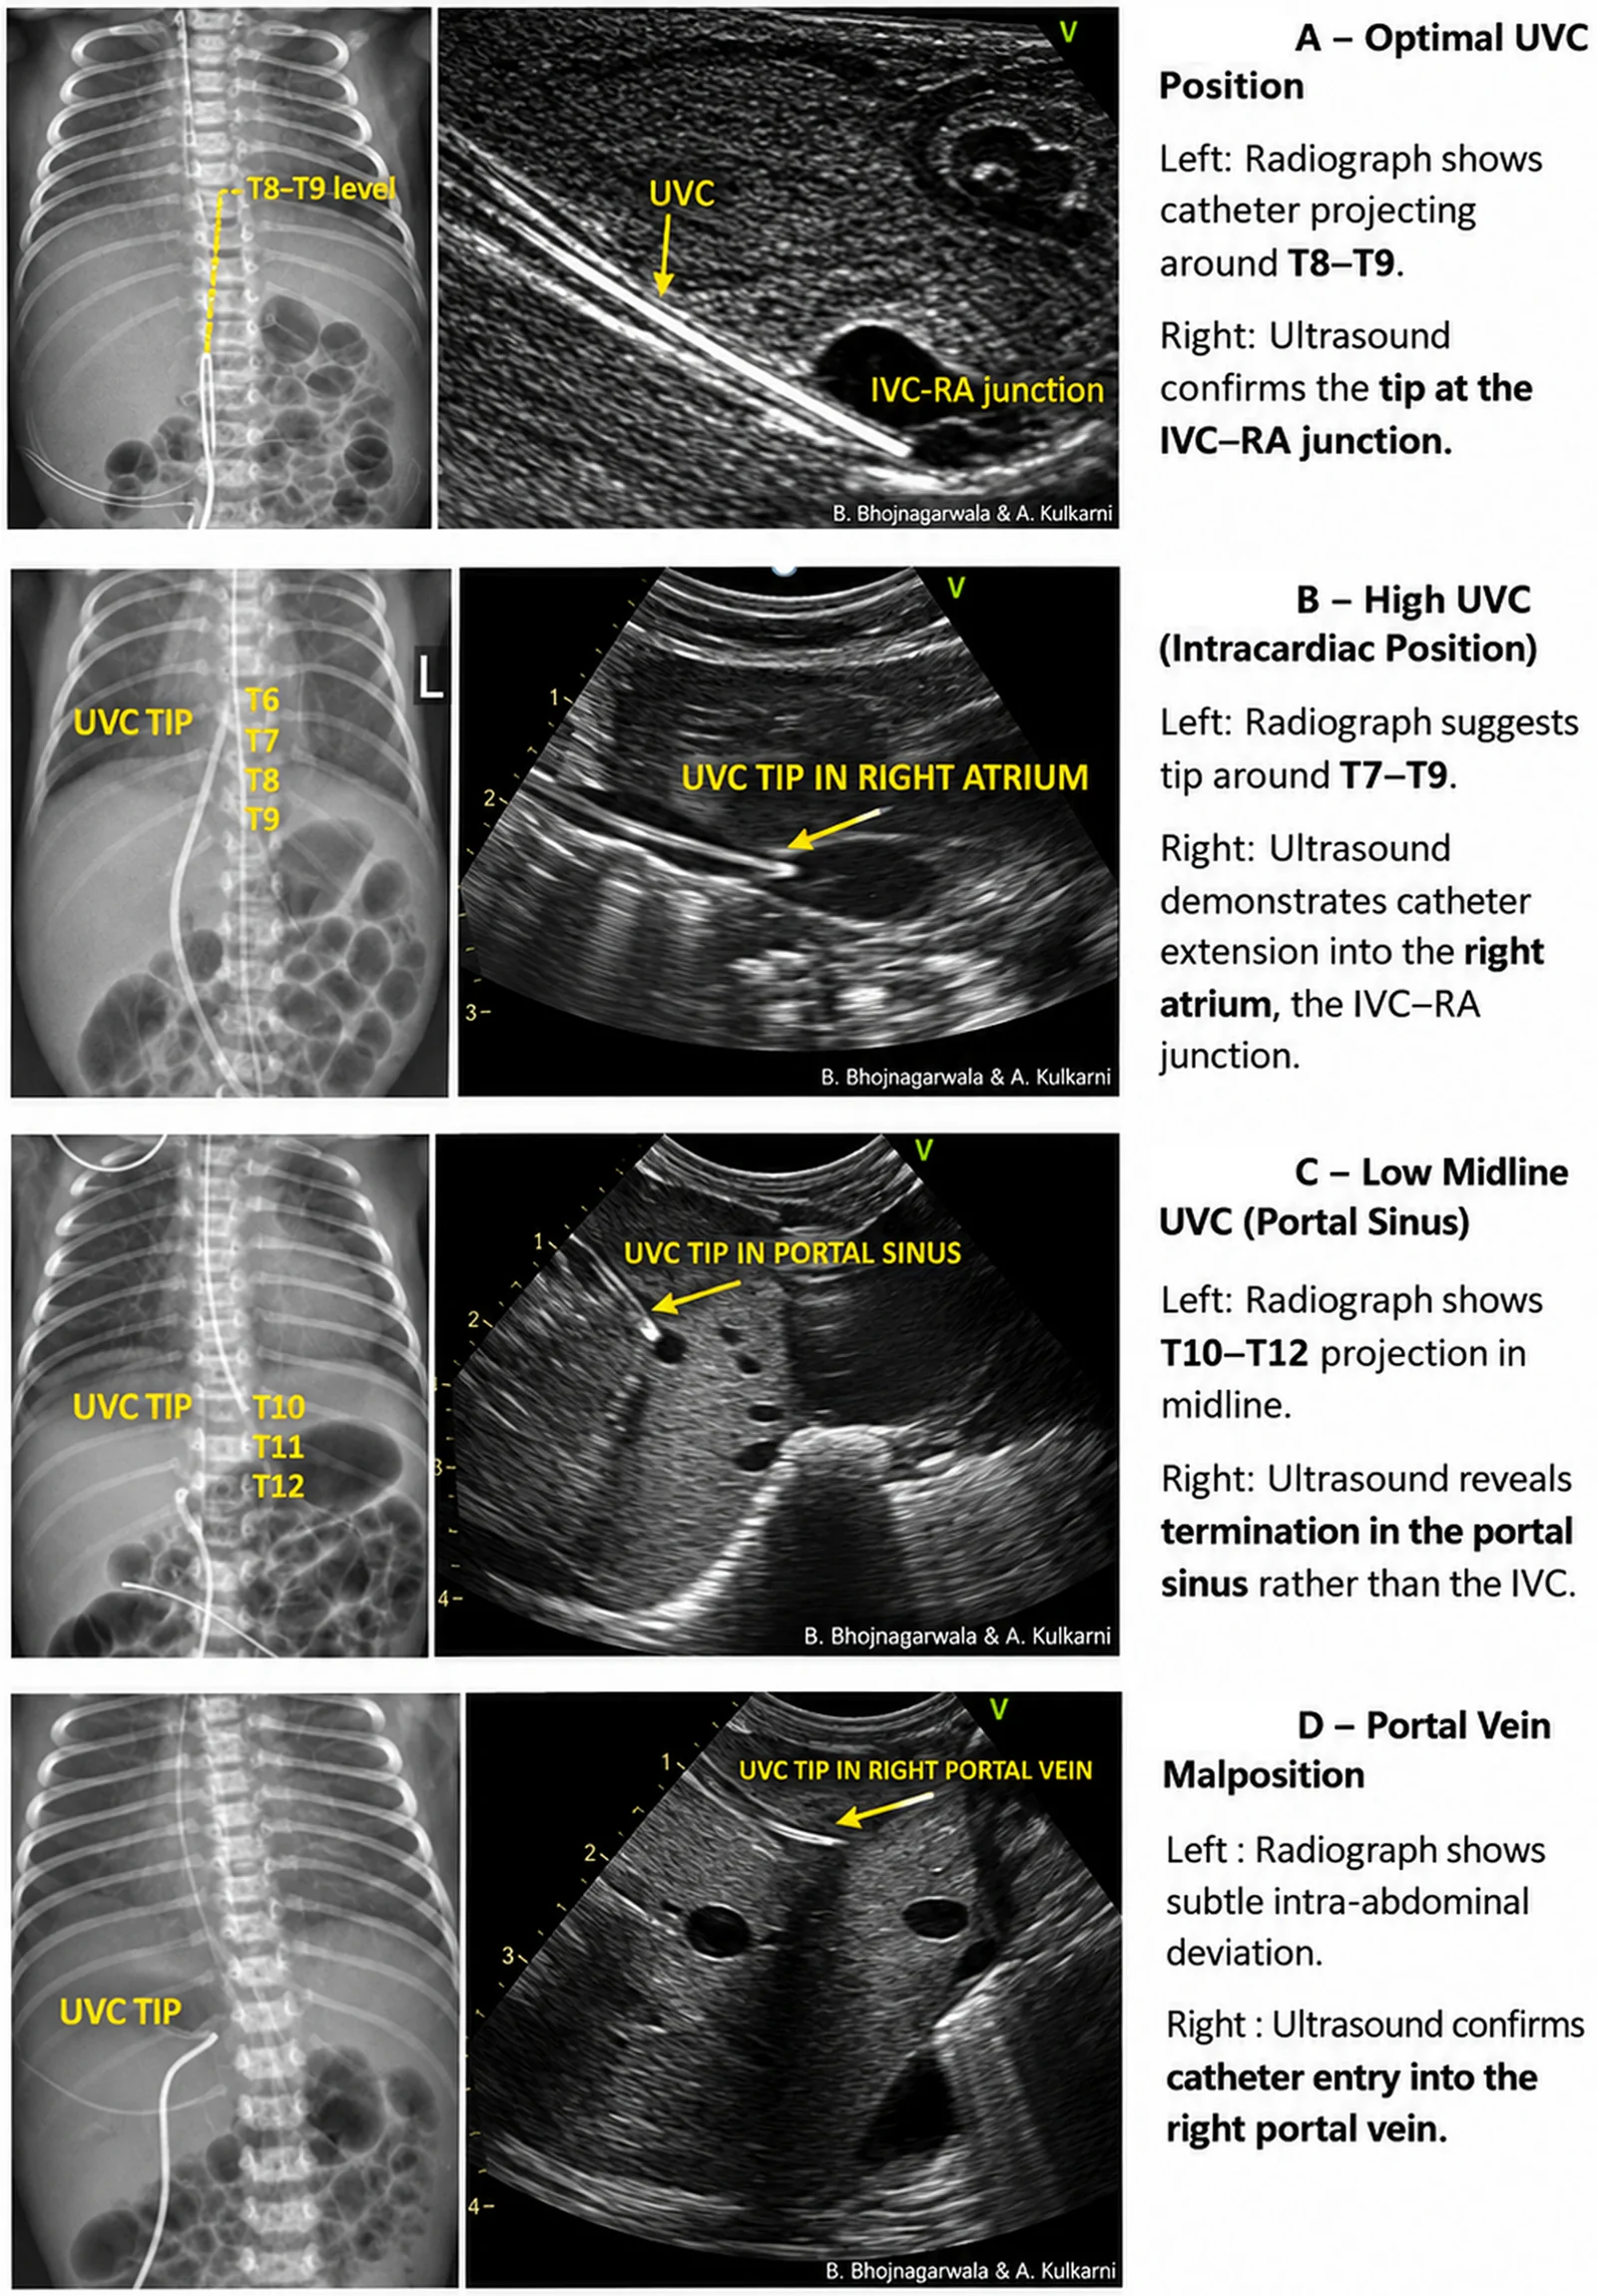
Radiographic versus ultrasound assessment of tip of UVC catheter
